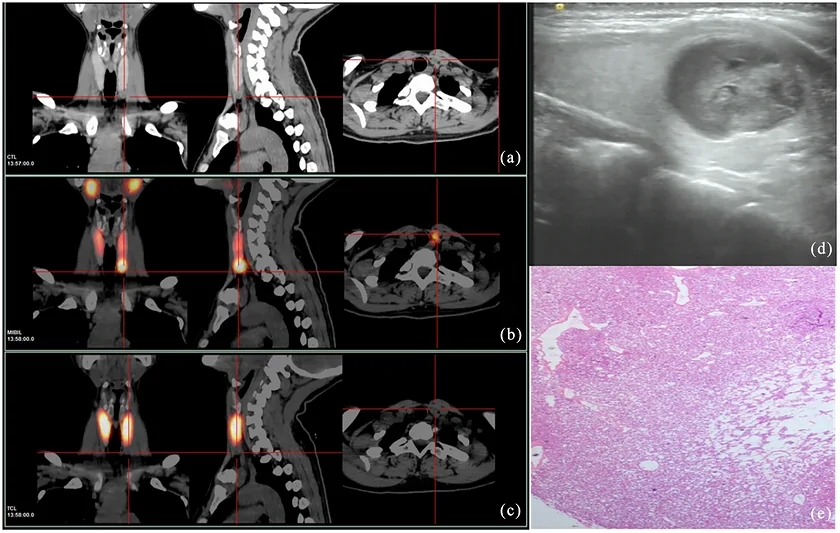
This patient suffered from dry mouth, dizziness, bone pain, and fatigue for more than 1 month due to unprovoked causes. Blood calcium: 2.71 mmol/L, PTH: 801.7pg/ml. Dual‐isotope SPECT/CT imaging: A round‐like soft tissue density lesion is visible on CT (a), with the lesion showing positive on ^99m^Tc‐MIBI images (b) and negative on ^99m^TcO_4_
